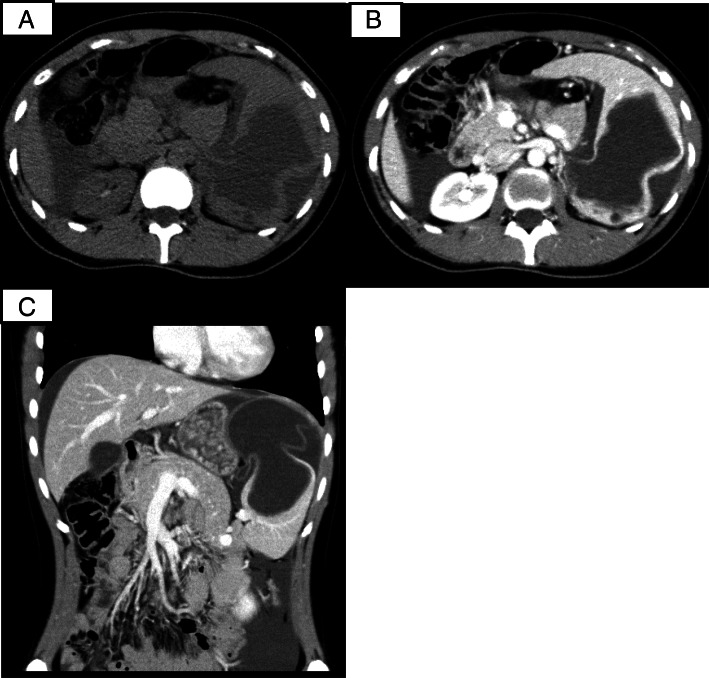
An abdominal CT scan showed a collapsed cystic lesion, measuring 12 cm × 12 cm × 8 cm, in the spleen and abdominal fluid in Morison’s pouch and around the liver and spleen (a). Moreover, an 8-mm cyst and a small collapsed cystic lesion were found posterior to the large cystic lesion on a contrast-enhanced CT scan (b). There was no evidence of contrast medium extravasation (c)
